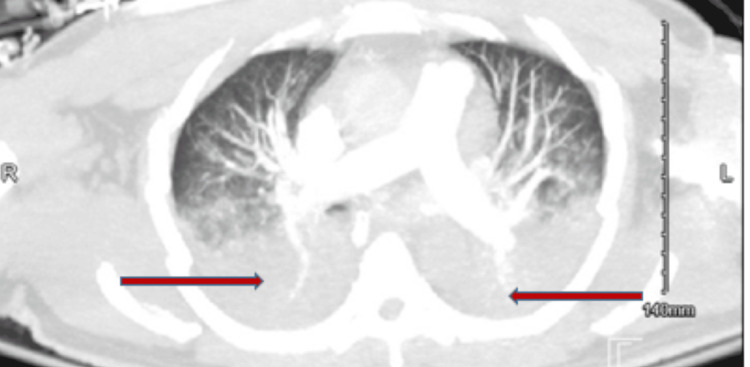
CT of the chest showing diffuse bilateral dense pulmonary infiltrates consistent with acute pulmonary edema and possible pneumonia CT: computed tomography
